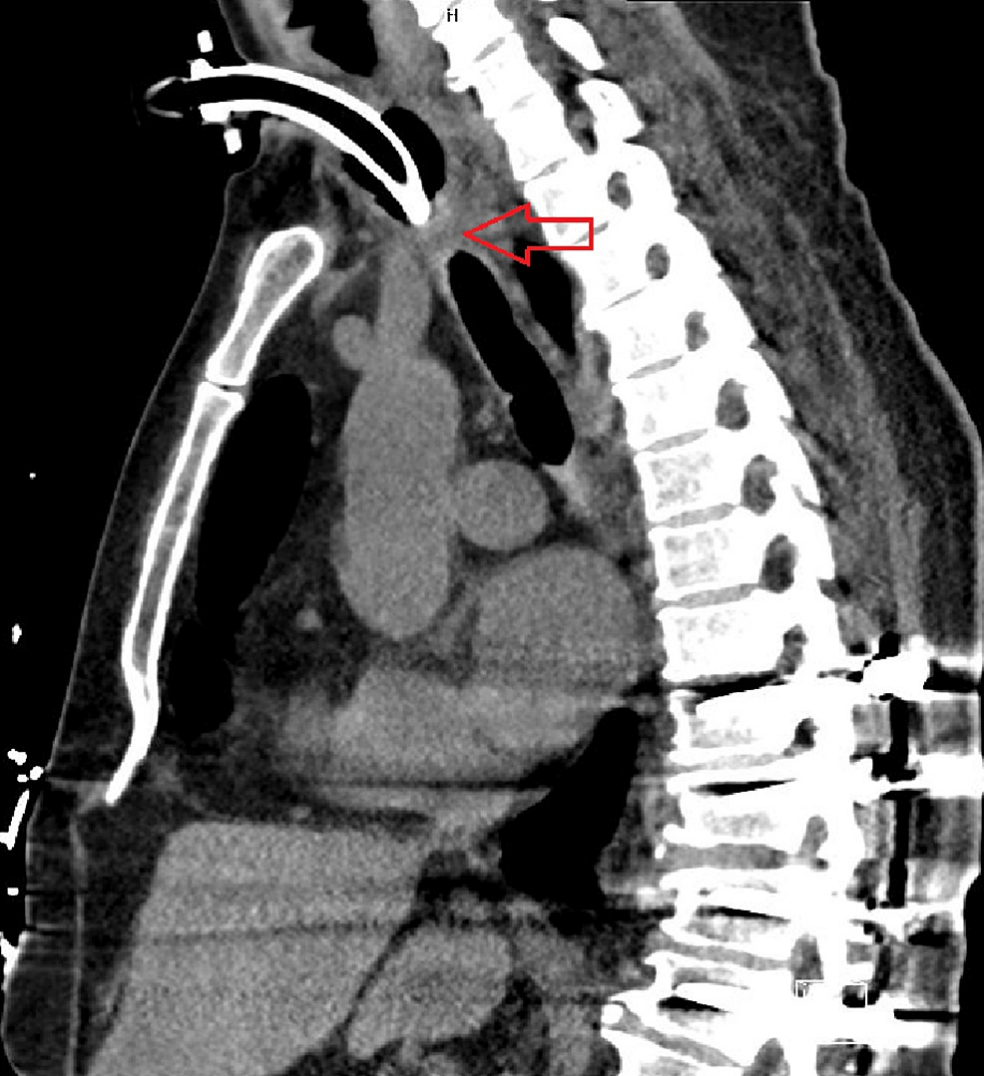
CT chest in sagittal view showing tracheal stenosis distal to tracheostomy tube
